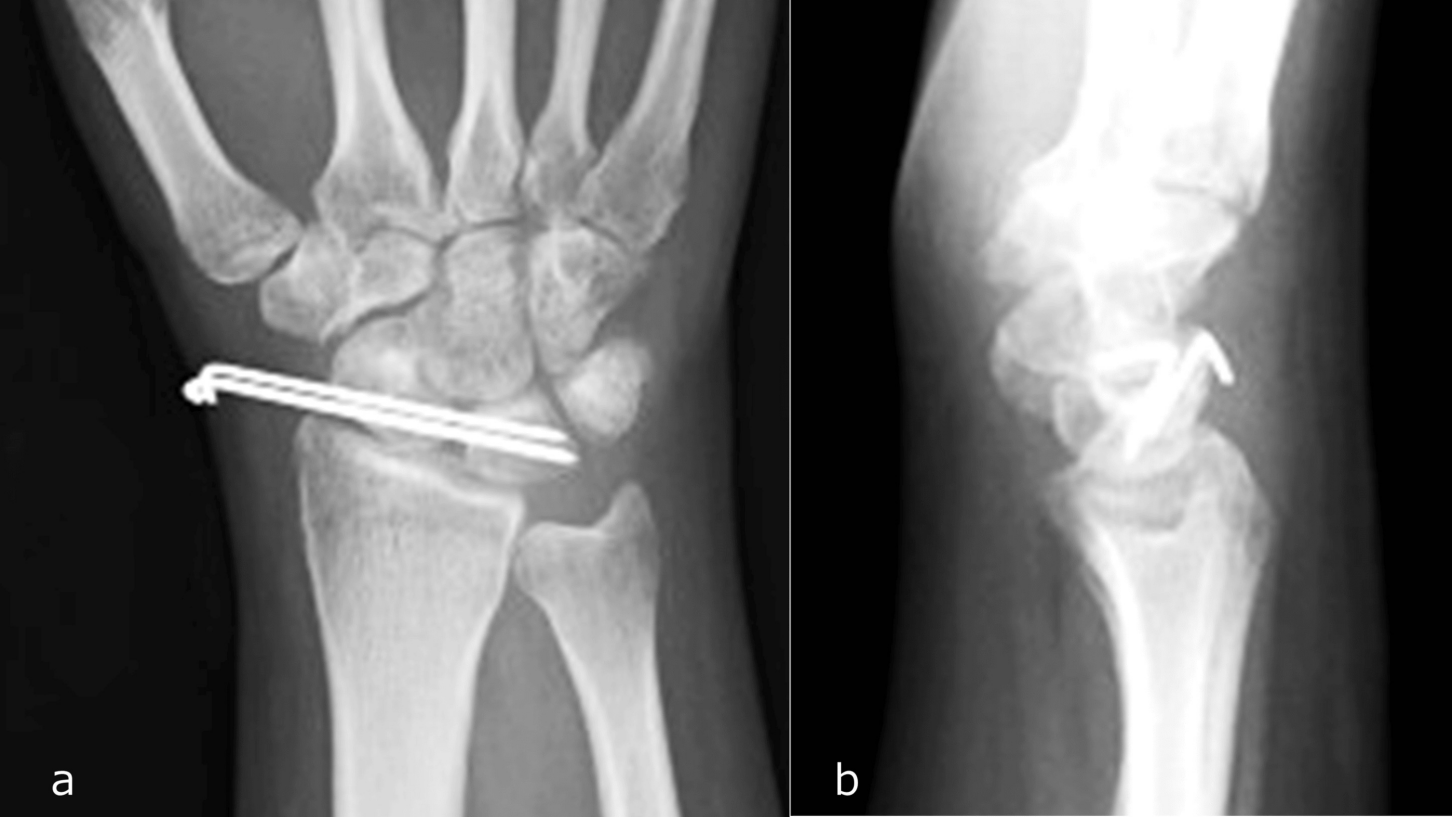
Postoperative X-ray following the initial surgery. a: Anteroposterior view. b: Lateral view.
